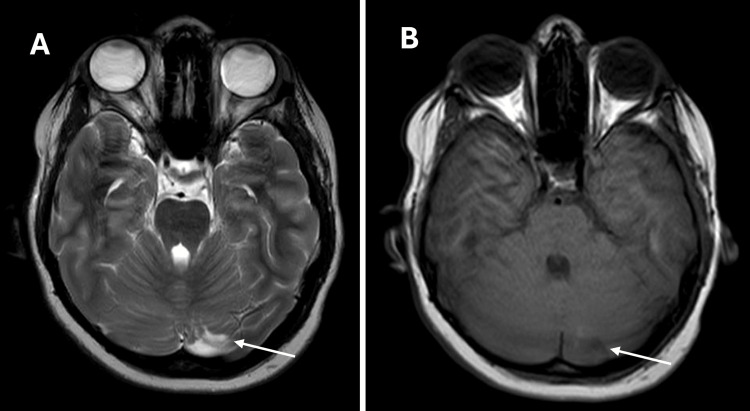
Follow-up non-contrast brain MRI demonstrating interval resolution of the left cerebellar abscess. (A) Axial T2-weighted image demonstrating marked reduction in size of the left cerebellar lesion with near-complete resolution of surrounding signal abnormality (white arrow). (B) Axial T1-weighted image showing no residual pathological signal, consistent with resolving abscess. No mass effect or hydrocephalus is present (white arrow). Contrast administration was declined by the patient's family; post-contrast assessment was, therefore, not performed. MRI, magnetic resonance imaging
